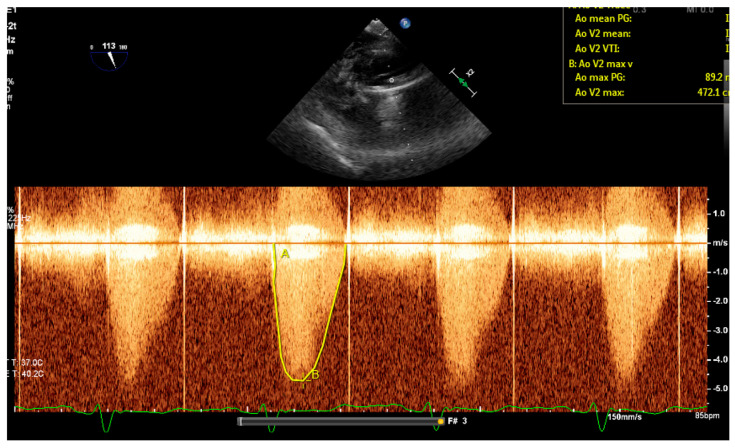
Transesophageal echocardiography Doppler prior to ultraslow low-dose alteplase infusion showing a mean aortic transvalvular gradient of 52 mmHg and aortic valve peak velocity of 472 cm/s, consistent with severe aortic stenosis.
